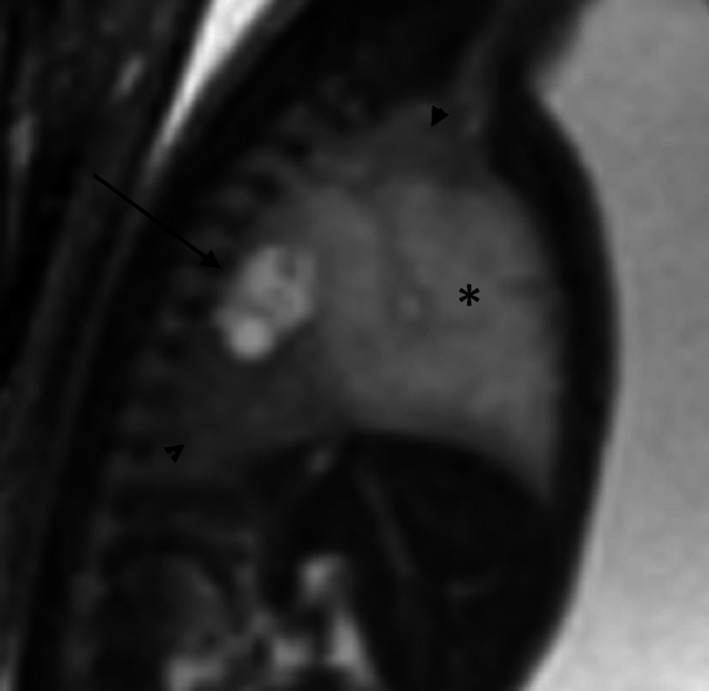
Right parasagittal SSFP MRI sequence of the fetal chest demonstrating the relationship of the cluster of small cysts (black arrow) with the mildly compressed normal lung (black arrow heads) and the region of T2 hyperintensity and overinflation (asterisk)
